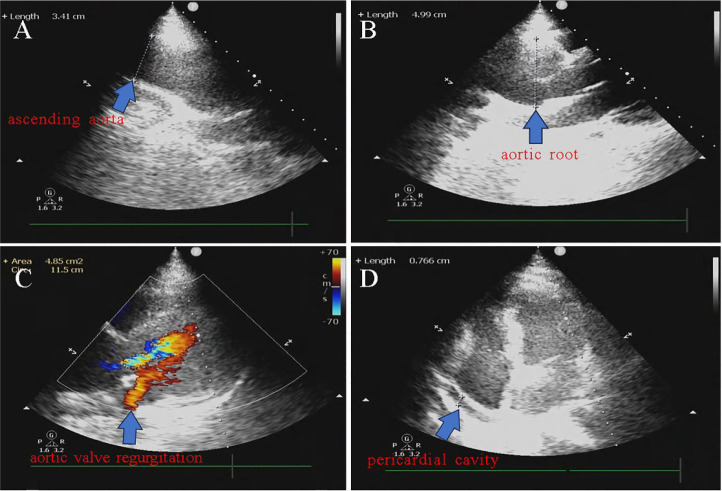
Echocardiography images. A) The inner diameter of the ascending aorta was
